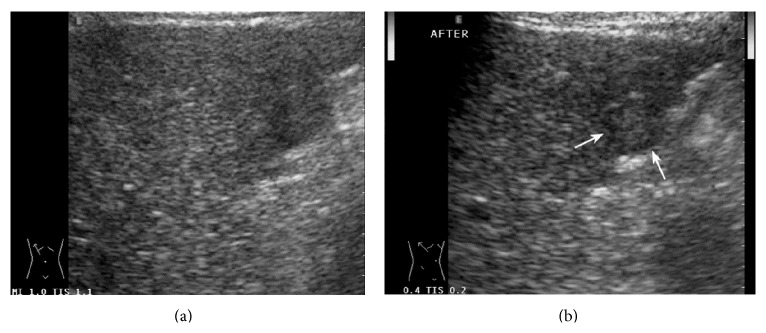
A 74-year-old man was found to have a liver tumor during the routine ultrasound examination. (a) US pre-CEUS showed an iso-echoic lesion with poor-defined margin in cirrhotic liver background. (b) US post-CEUS showed that the lesion had clearer border and a hypoechoic halo (↑) at 8 minutes after contrast agent injection. The CEUS pattern was atypical for this lesion. The diagnosis result of HCC was improved by US post-CEUS.
